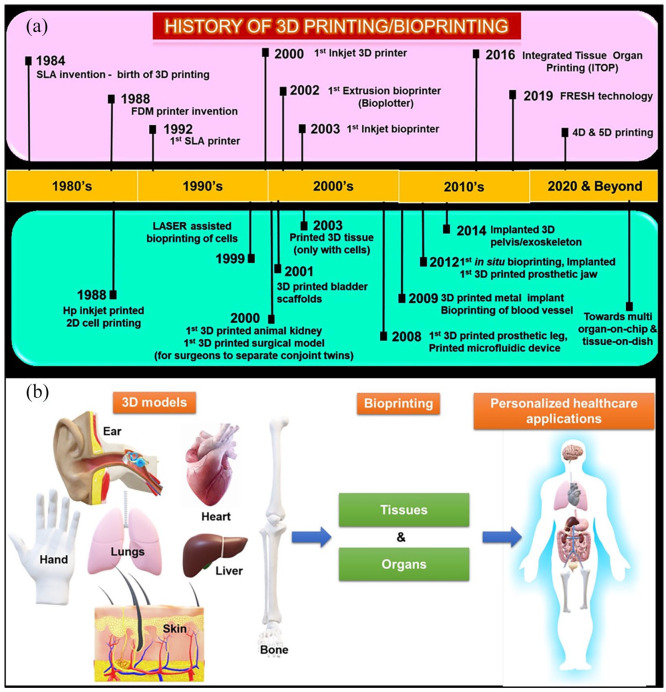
(a) Chronological history of 3D printing/bioprinting in potential biomedical applications, (b) imagined future of 3D bioprinting—3D models obtained through CT or MRI images and computationally redesigned according to the personalized requirements.
